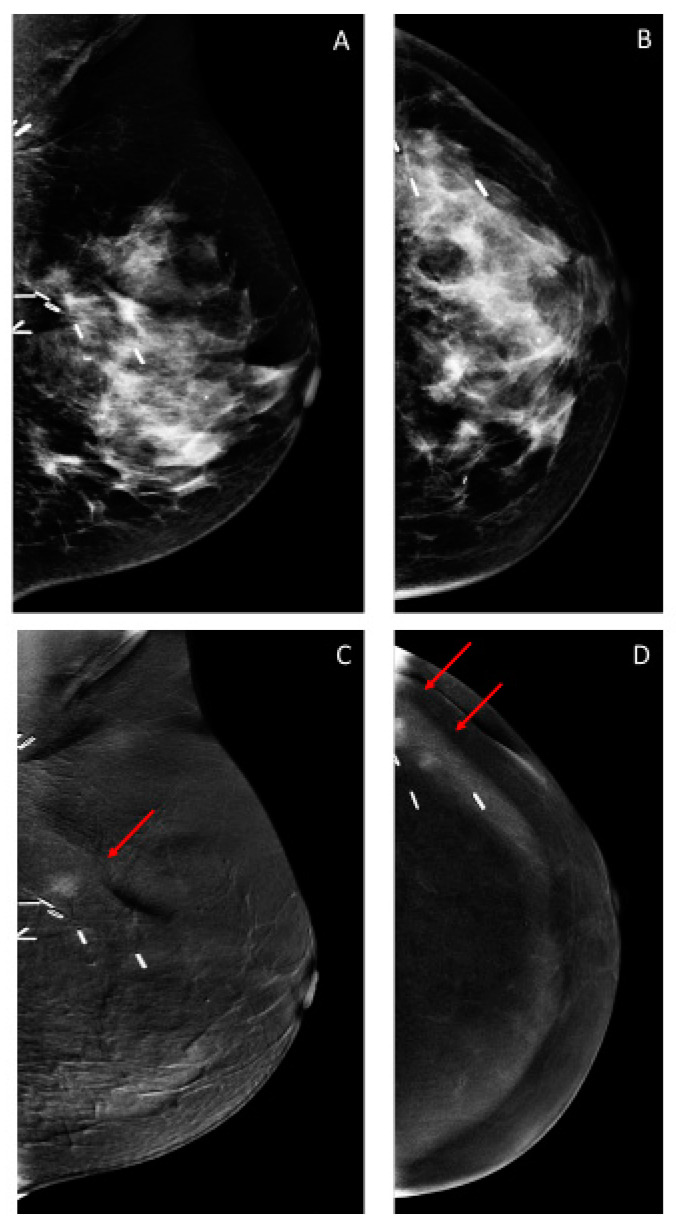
Forty-nine-year-old woman treated with left breast conservation surgery 3 years prior for unifocal invasive ductal carcinoma (IDC) and ductal carcinoma in situ (DCIS). (A,B) No suspicious findings on low-energy images. (C,D) Iodine images show two sub-centimeter areas of non-mass enhancement immediately superior and lateral to the surgical clips at the lumpectomy bed (arrows); one lesion is obscured on the mediolateral oblique (MLO) view by an overlying surgical clip. Two sonographic correlates were identified and biopsied, both yielding IDC. The patient was then treated with a mastectomy.
